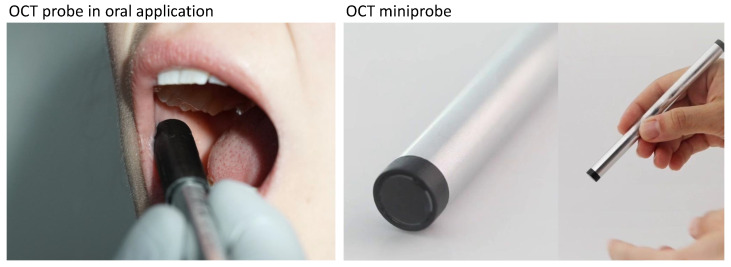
Application of the OCT probe in the human oral cavity in vivo by means of OCT imaging of the buccal mucosa of a healthy subject for demonstration. The OCT miniprobe is covered with a dental disposable plastic cover (X-ray Cover, Henry Schein Inc., Melville, NY, USA) fixed by an one-use cover sleeve (see left). Customized OCT miniprobe in the overall and detailed view of the distal end of the probe without disposable dental cover for single use in the oral cavity (see right).
